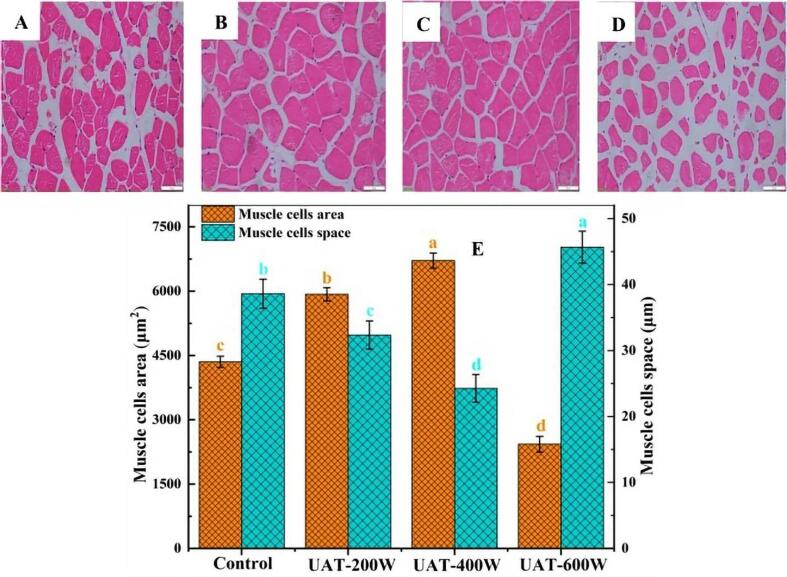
Microstructure of frozen white yak meat with different thawing treatments. UAT, ultrasound-assisted immersion thawing at different ultrasound powers (200, 400 and 600 W) [53].
